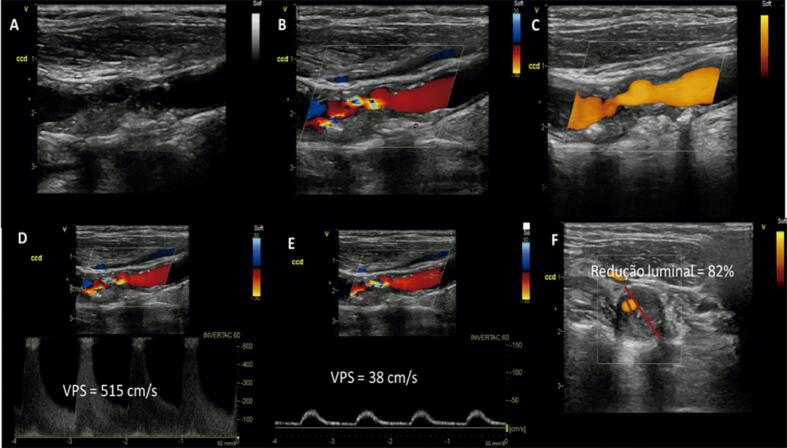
Estenose da artéria carótida comum. A) Modo B; B) Doppler colorido; C)* Power *Doppler; D) Elevação do VPS no ponto da estenose da artéria carótida comum; E) VPS pré-estenose; F) Corte transversal com importante redução do lúmen residual. VPS: velocidade do pico sistólico.
